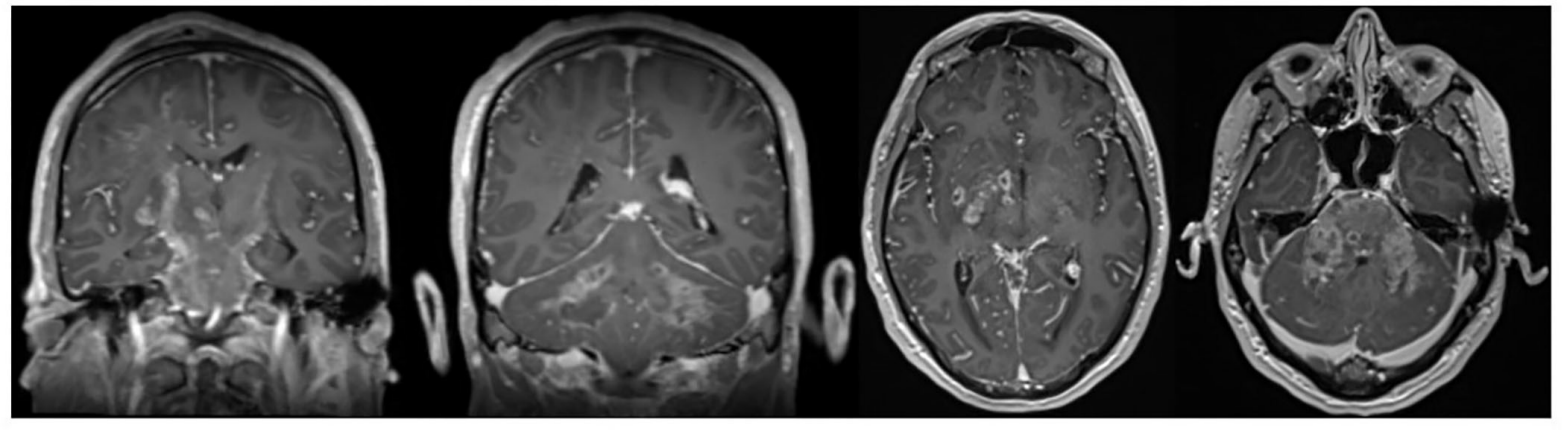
Axial and coronal contrast-enhanced T1-weighted images showing typical rim-enhancing microabscesses spreading along white matter tracts.
